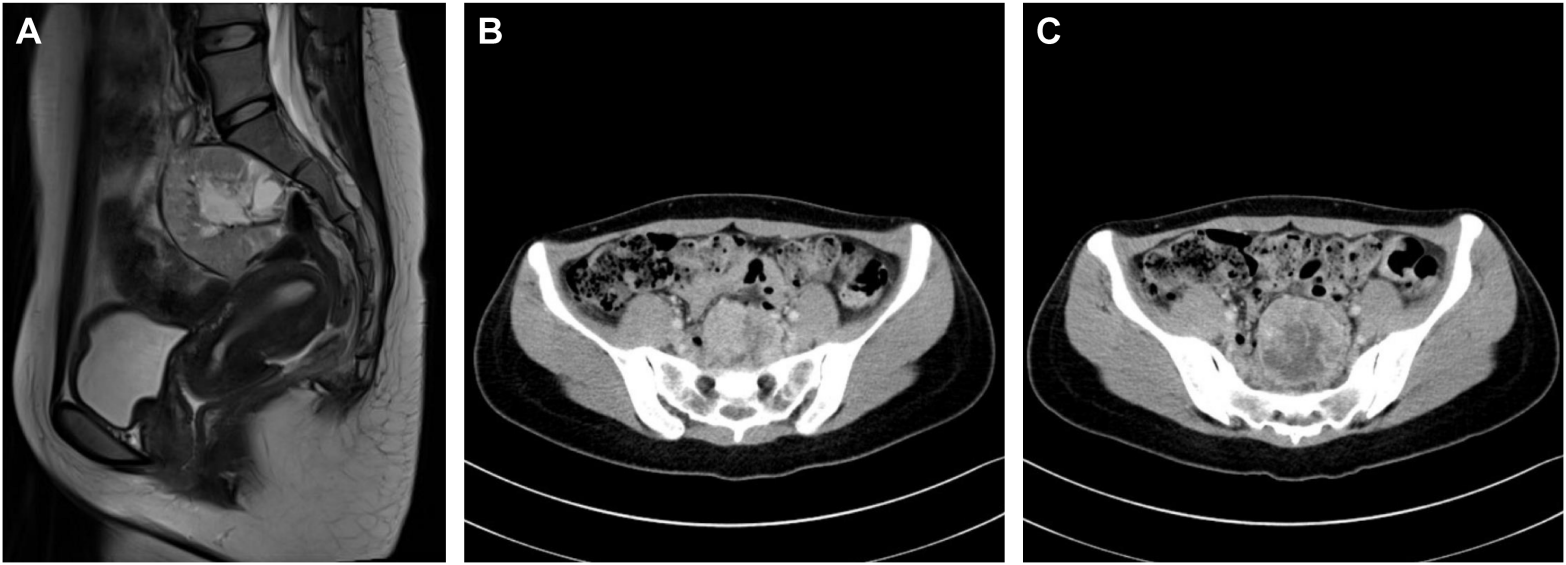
Imaging of a pelvic PGL (A) MRI of the sagittal position of the PGL. (B) The upper part of the PGL was close to the sacrum. (C) The maximum cross-section of the PCC was about 6.0cm×6.1cm.
